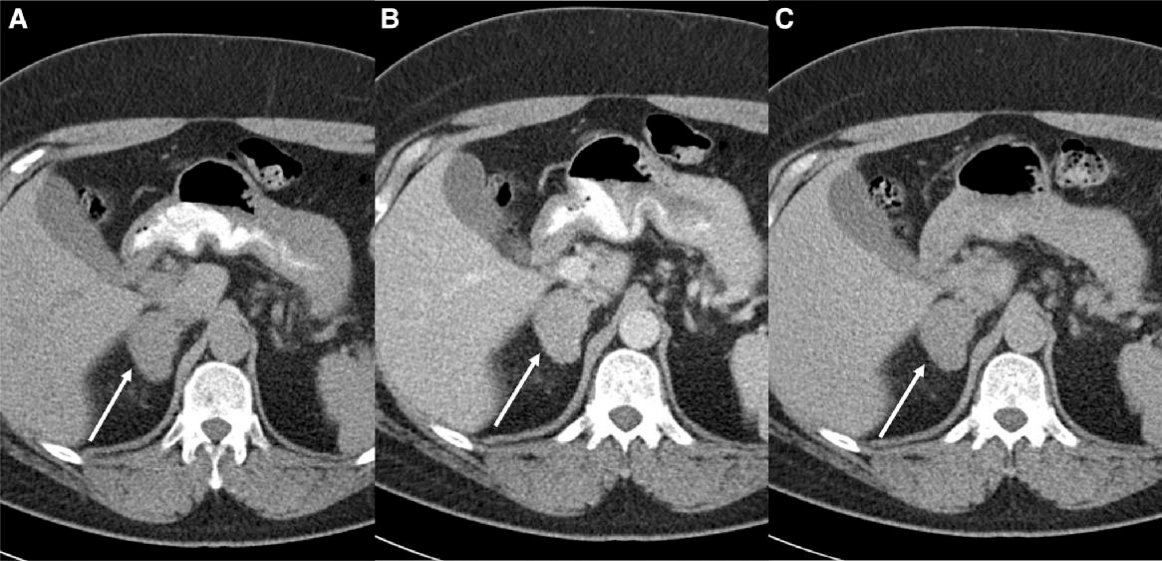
Unenhanced axial CT from 2017 demonstrates a 42-mm right adrenal mass with attenuation of 32 HU (A). Homogenous enhancement was demonstrated on portal venous phase CT (B) with marked washout on delayed 15-minute imaging (C). Absolute contrast washout of adrenal mass is 78% and relative contrast washout is 36%.
